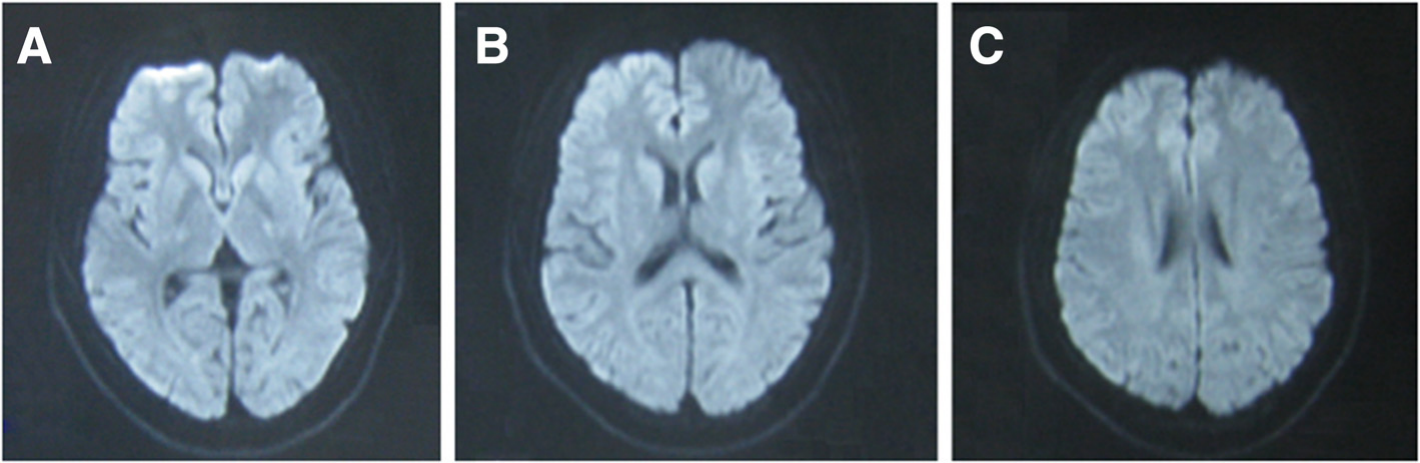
The third MRI after two mouths of onset. Diffusion-weighted MRI after two months of onset by which time the patient has no repeated neurological attracks, shows nearly complete resolution of the white matter changes (A, B and C).
